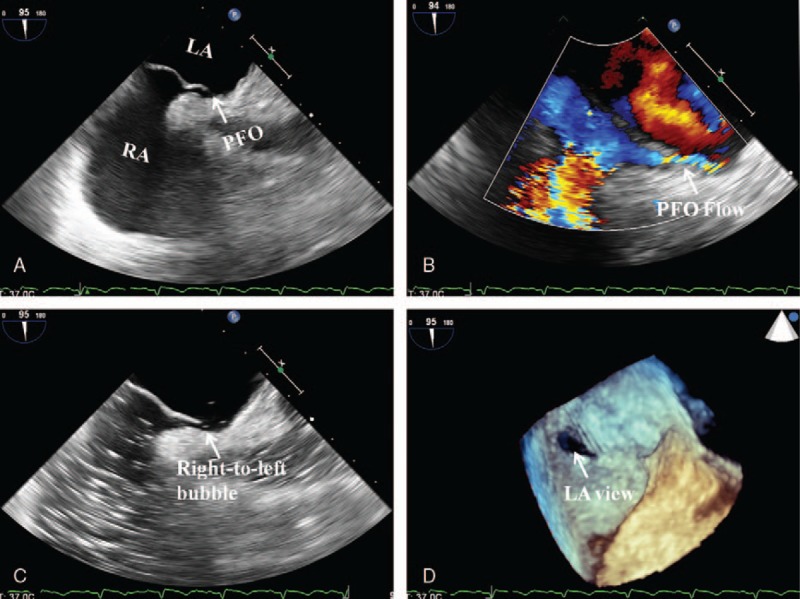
Transesophageal echocardiography (TEE) confirming the PFO (arrow). (A) The 2-dimensional TEE in the biatrial view detected a separation between the primum and secundum atrial septum. (B) Color Doppler demonstrated bilateral but mainly right-to-left flow by decreasing the color gain and wall filtration. (C) Contrast TEE revealed right-to-left shunt after the contrast agent (6 mL of 1% injection vitamin B6 and equal volume of 5% sodium bicarbonate solution) was administered through the dorsal vein of right hand. (D) Real-time 3-dimensional TEE confirmed the PFO. LA = left atrium, PFO = patent foramen ovale; RA = right atrium.
